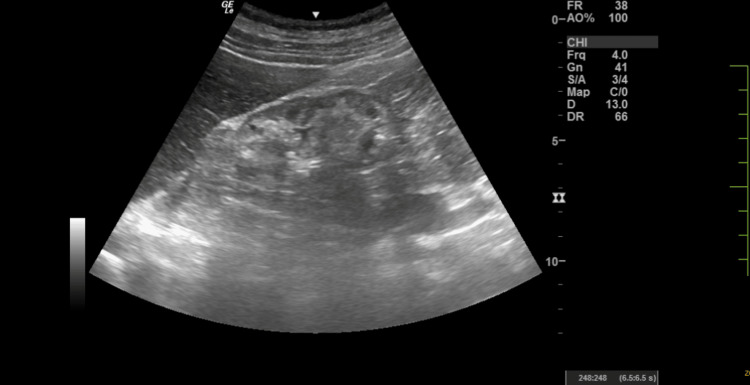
Ultrasound abdomen showing the right kidney The right kidney appears small in size and corticomedullary differentiation poorly maintained
